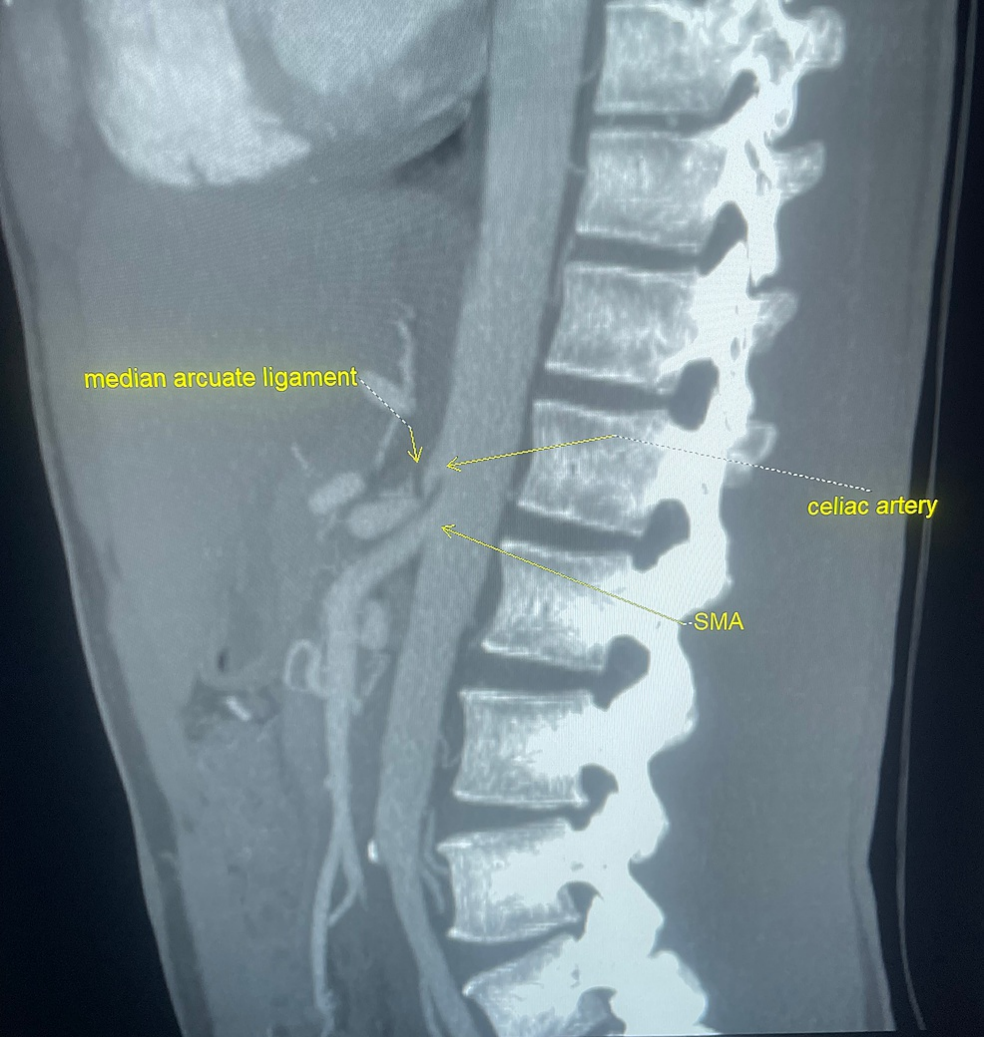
Computed tomography angiography showing celiac artery stenosis due to overlying median arcuate ligament with post-stenotic dilation. SMA = superior mesenteric artery.
